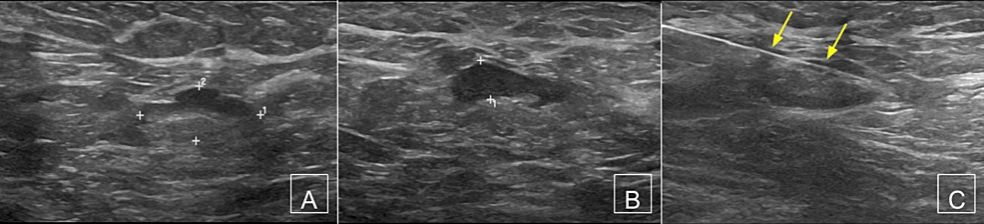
Suspicious axillary lymph node on sonography (A and B) Long-axis grayscale images through the 1.6 × 0.7 × 1.5 cm axillary lymph node demonstrated suspicious focal cortical thickening, prompting a biopsy. (C) An ultrasound-guided biopsy revealed metastatic ductal carcinoma (arrows = needle).
